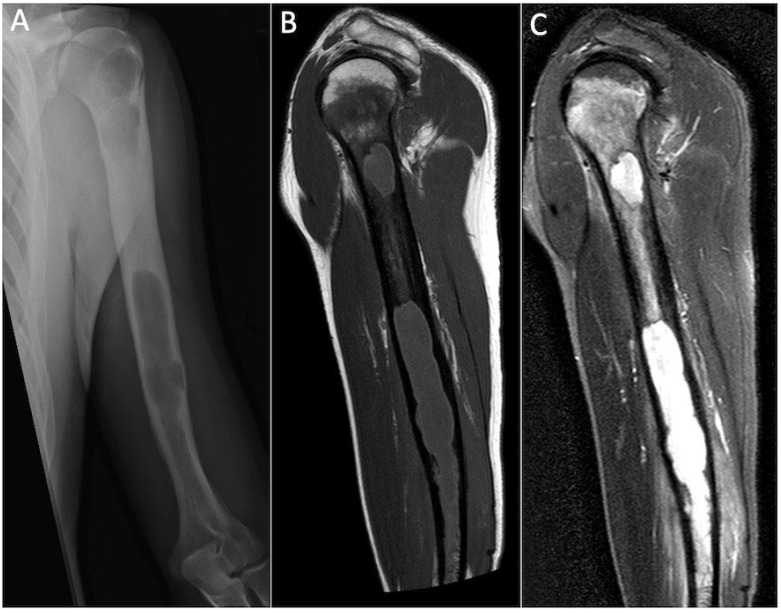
Humeral anteroposterior radiograph (A), sagittal T1-weighted (B) and STIR (C) MRI, demonstrating large intraosseous abscesses with the penumbra sign and marked surrounding intramedullary oedema, with free fat globules present, more so at the proximal metaphysis.
